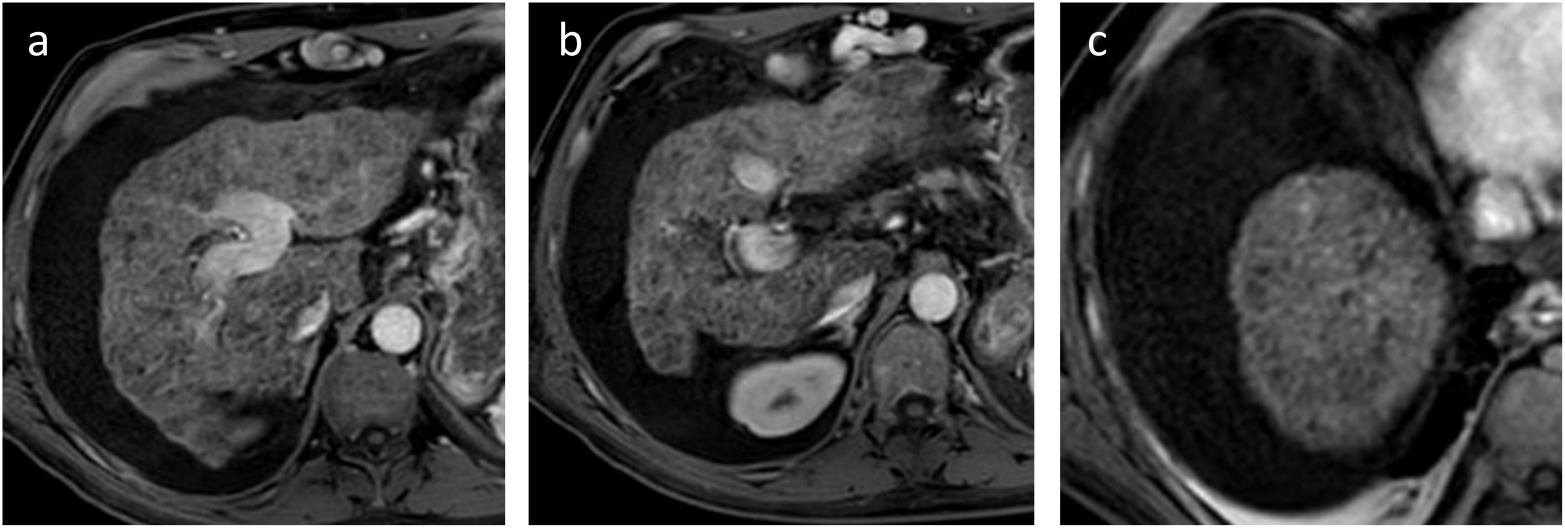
Last follow-up MRI before LT showing complete disappearance of the hepatic lesions, associated with ascitic decompensation (sequence T1 with injection). (A) Main lesion in the left lobe; (B) segment IV and (C) hepatic dome.
